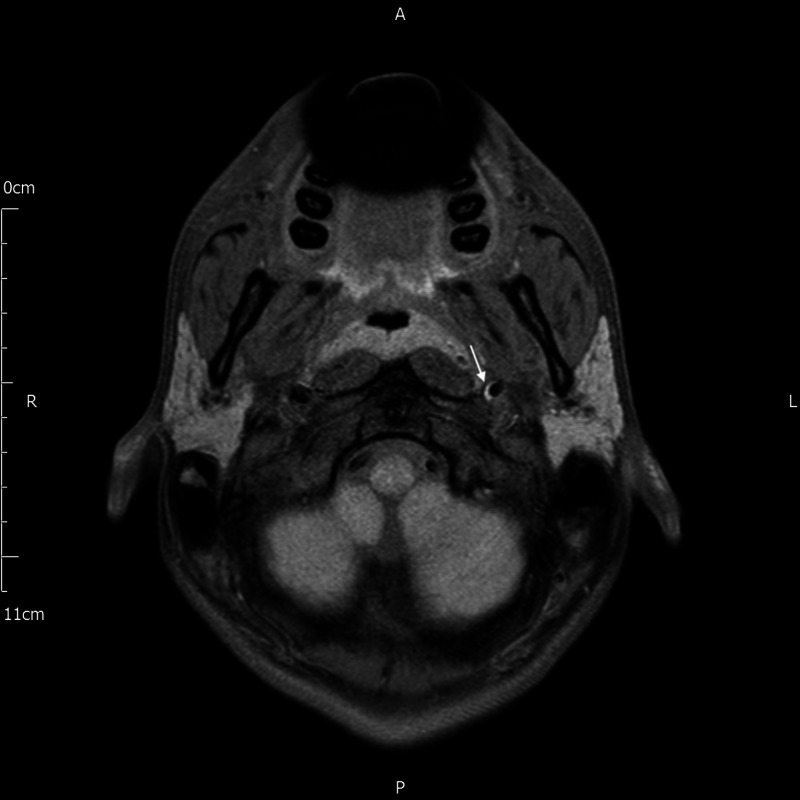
MRI scan with the white arrow highlighting crescent T1 hyperintensity of the medial wall of the left internal carotid artery, suggestive of an intramural haematoma
